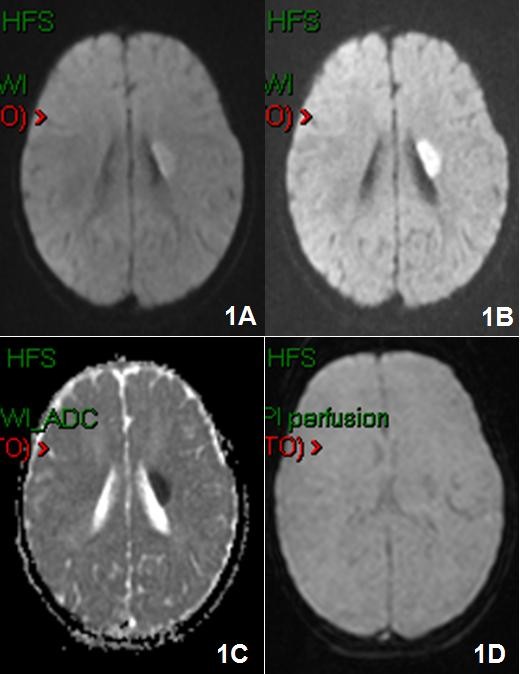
Brain MRI with Gd-DTPA was performed and showed the presence of an hyperacute ischemic lesion of about 2 cm of diameter, in the left lenticular nucleus extended to the internal capsule 1A), 1B), 1C) in DWI and 1D) perfusion weighted sequences.
